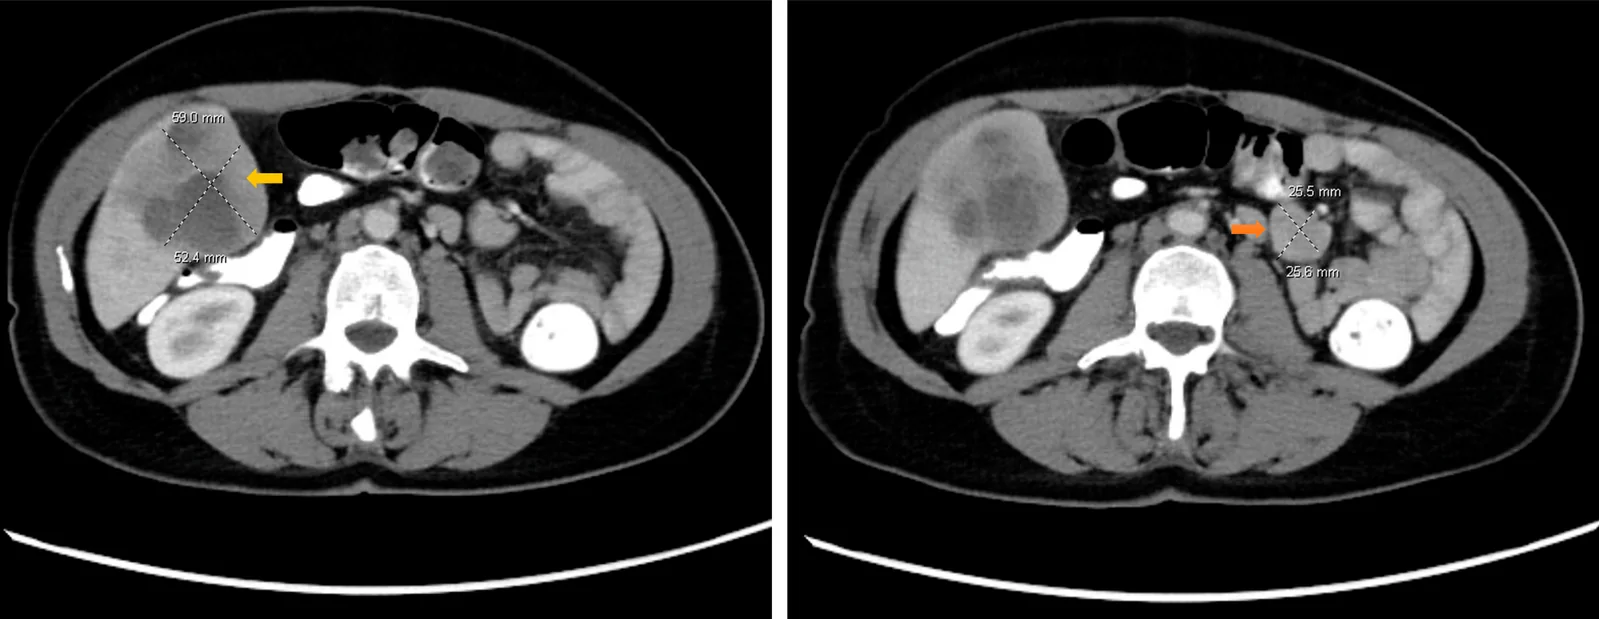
Findings on Computed Tomography of Abdomen Computed tomography of abdomen demonstrates a necrotic mass in the inferior right hepatic lobe measuring approximately 5.2 × 6.0 cm (yellow arrow) and a mesenteric mass in the left hemiabdomen measuring approximately 2.6 × 2.6 cm (red arrow) suspicious for metastatic neuroendocrine disease.
